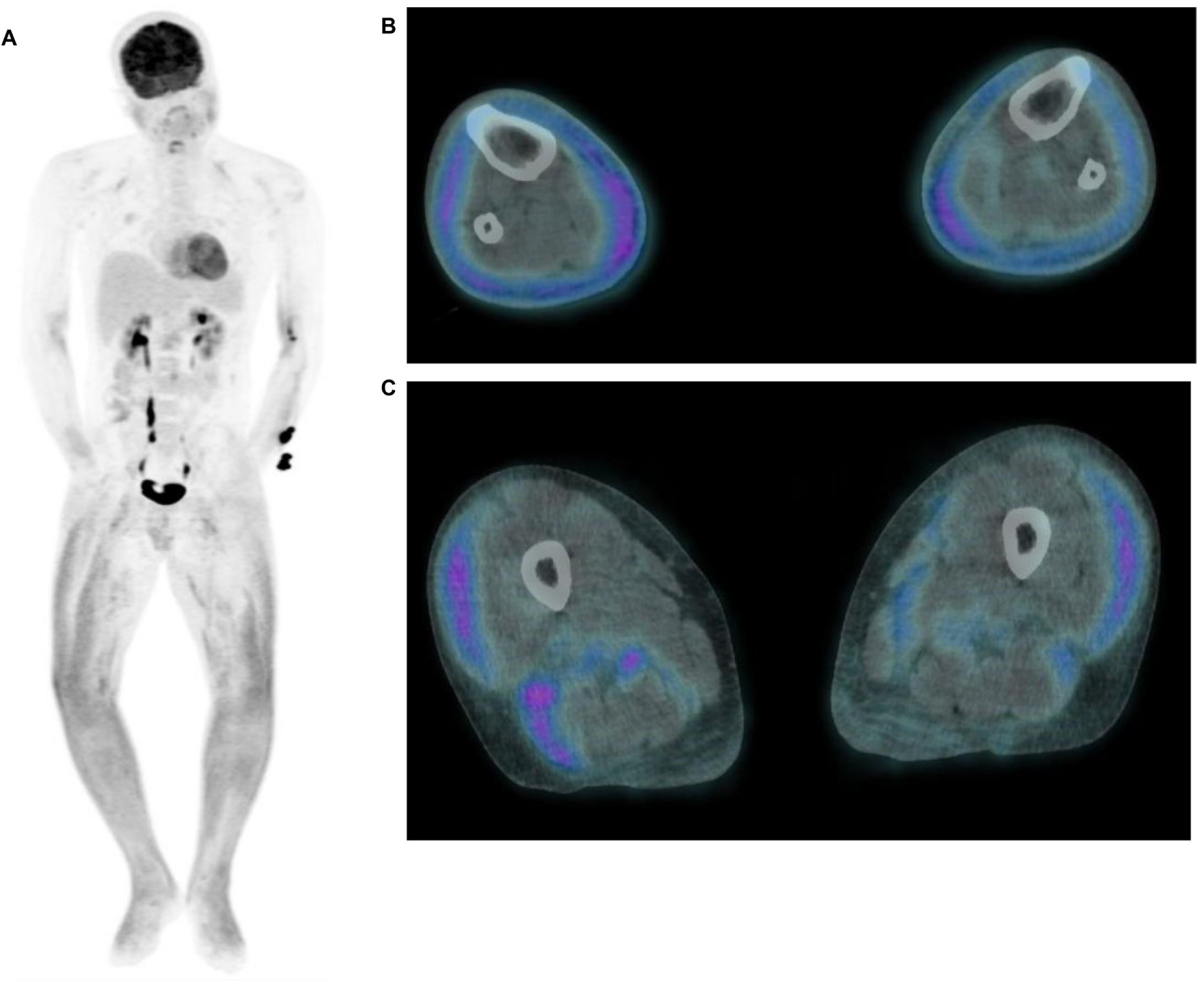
^18^F-FDG-PET/CT imaging of a 29-year-old male patient with IgG4-related lower limb fasciitis. Maximum intensity projection images of the ^18^F-FDG-PET/CT demonstrate diffuse tracer uptake in both lower limbs (A). No classical IgG4 disease sites or any other FDG-avid disease are demonstrated. Fused axial slices of the ^18^F-FDG-PET/CT demonstrate increased avidity at the fascial planes within the anterior and posterior compartments of the lower legs (B); these changes also extend to involve the upper legs (C). ^18^F-FDG-PET/CT, ^18^F-fluorodeoxyglucose-positron emission tomography/computed tomography; IgG4, immunoglobulin G4.
